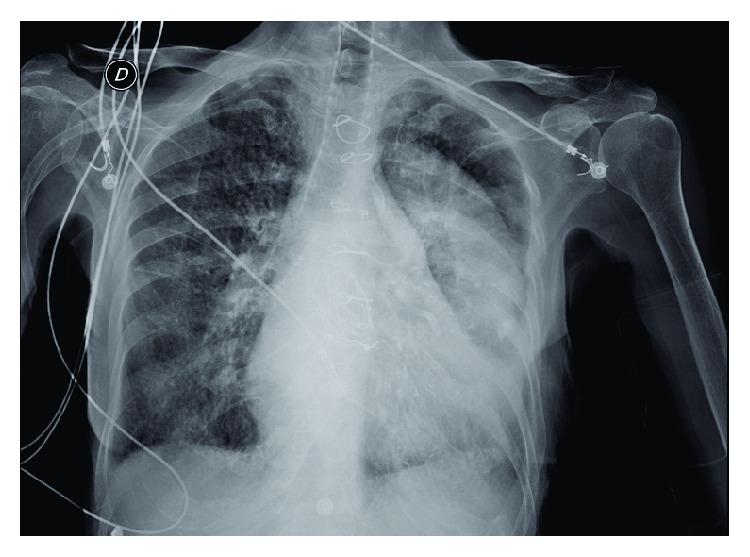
Chest X-ray showing pleural effusion.
